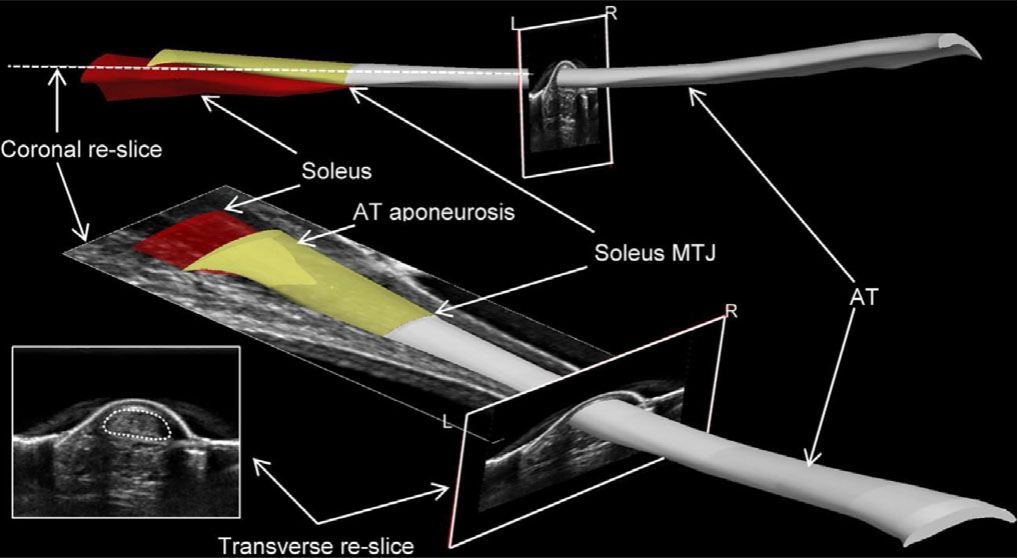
Freehand three‐dimensional ultrasound (FUS) reconstruction of the Achilles tendon (AT). Three‐dimensional ultrasound image reconstructions were created in Stradwin using manually digitized cross‐sections (dotted white lines within inset) of the tendon segmented at approximately 10‐mm to 15‐mm intervals along the length of the tendon^101^.
